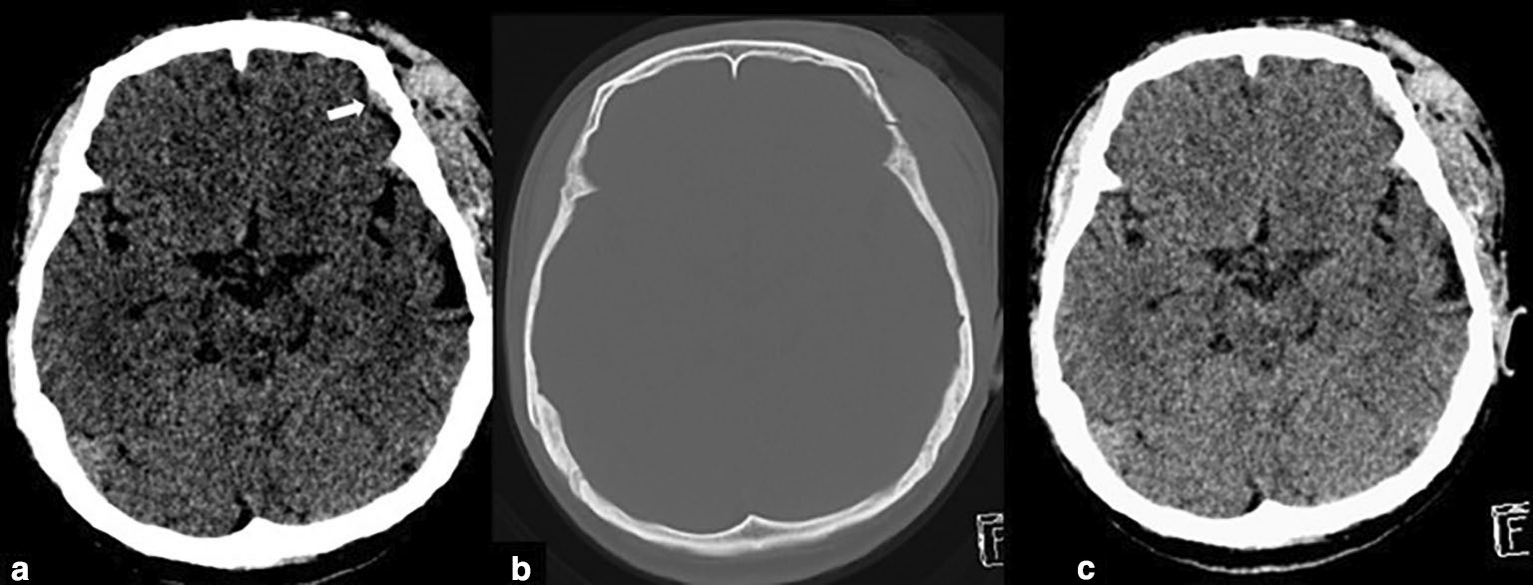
The CT scan of the brain shows a small left frontal convexity EDH (white arrow) in narrowed window setting (a) and associated skull fracture in the bone window setting (b). The post-processing image reveals that the EDH has not been annotated by the algorithm c).
